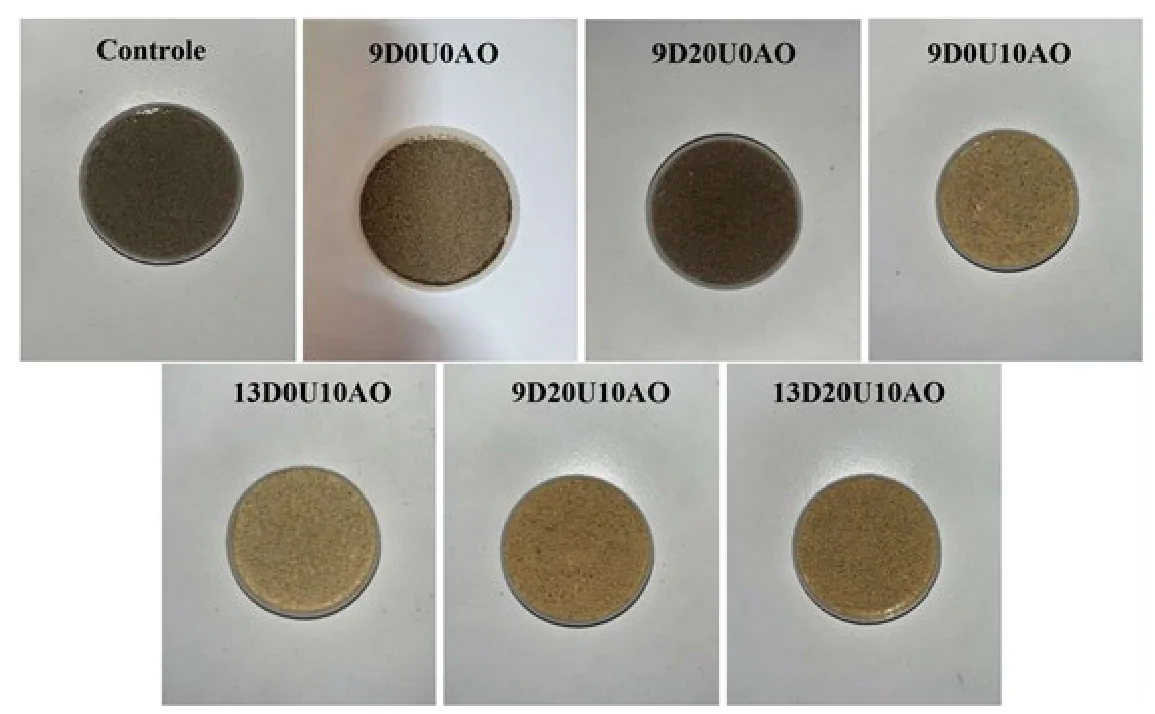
Plantain flour. The abbreviations D, U, and AO indicate the distance between the radiation source and the sample, the ultrasound application time, and the oxalic acid concentration, respectively.
